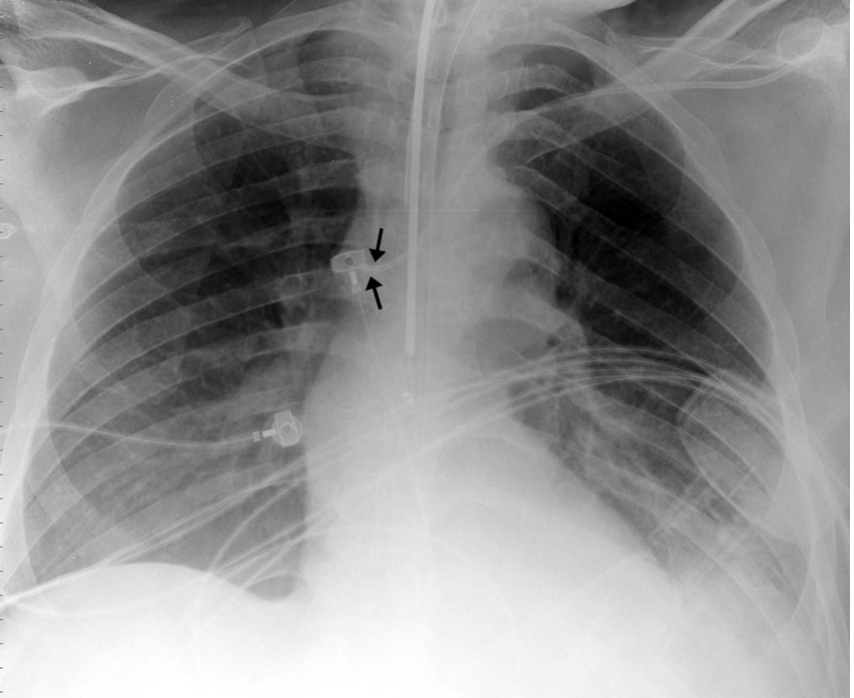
Chest radiograph showing the central venous catheter abutting the superior vena cava.
